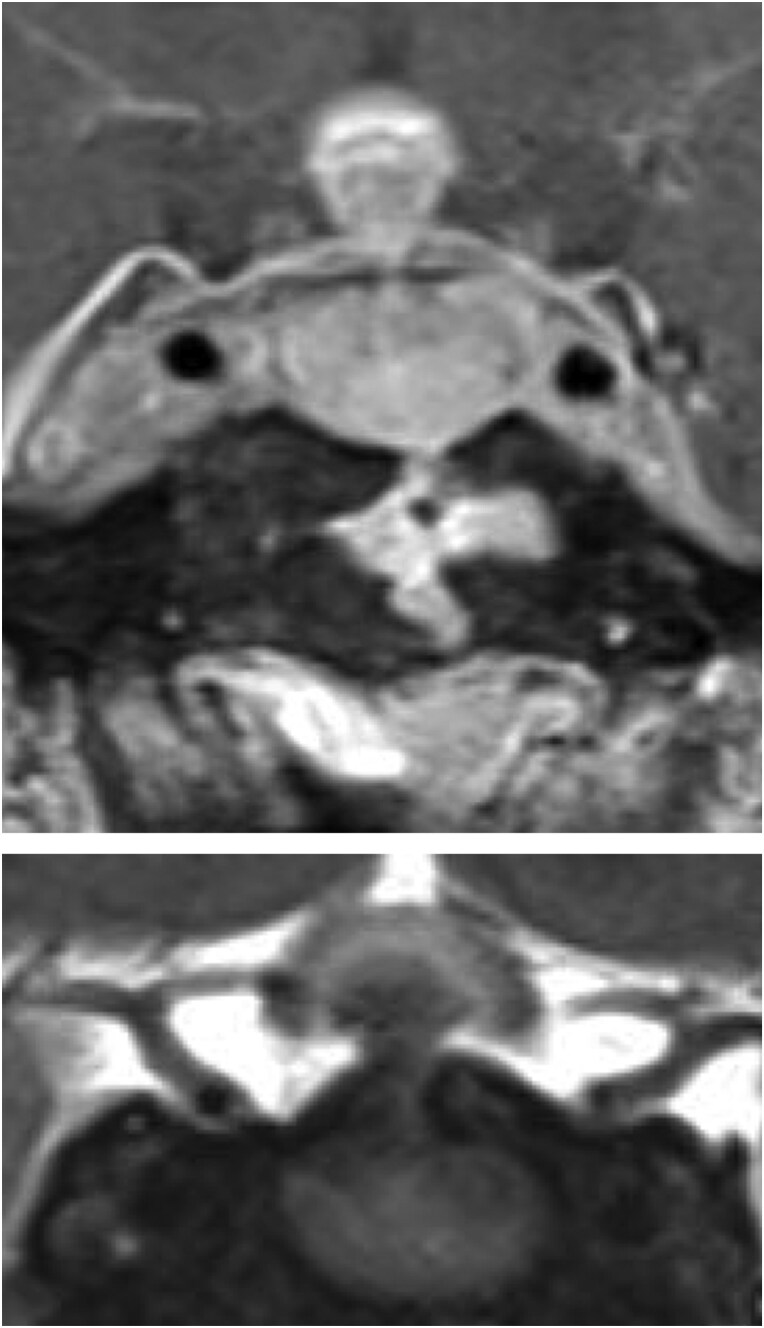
IgG4 hypophysitis—imaging. Left, postcontrast T1 pituitary magnetic resonance imaging coronal. There is infiltration of the pituitary gland, the infundibulum, and bilateral cavernous sinuses. The pituitary stalk is thickened with mass effect and upward bowing of the optic chiasm. Right, Coronal T2 weighted MRI shows associated mass effect and compression of the optic chiasm.
